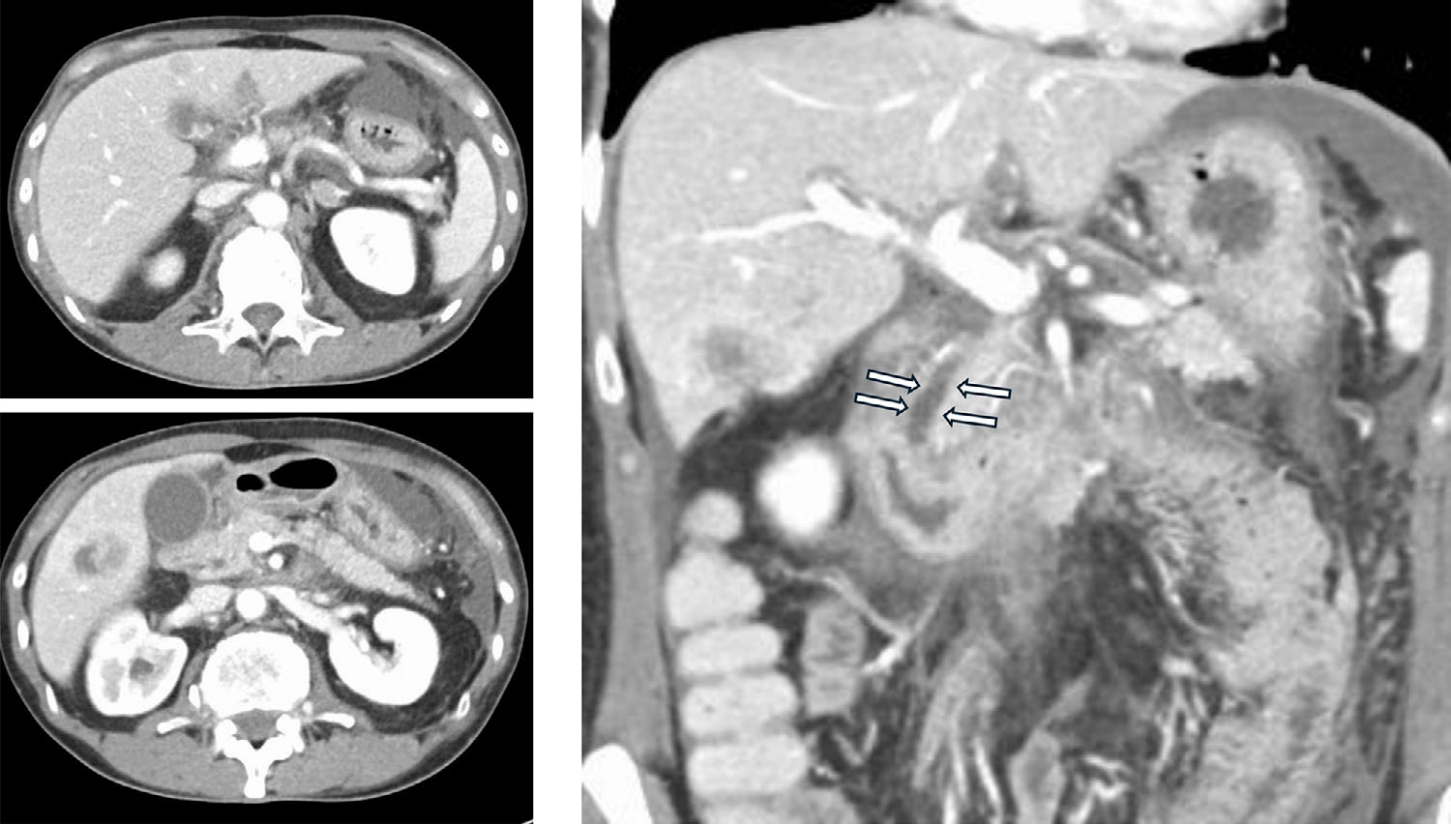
Contrast‐enhanced computed tomography (CT) revealed multiple liver metastases; no biliary ductal dilatation or intraductal masses were seen.
